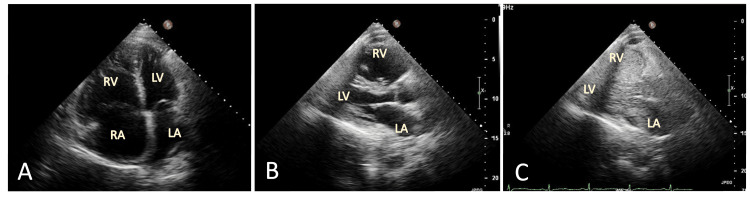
Initial TTE evaluation (A) Apical 4-chamber view. (B) Parasternal long axis view. (C) Demonstrating markedly enlarged right heart structures with marked right-to-left shunting of agitated saline. TTE: transthoracic echocardiogram; RV: right ventricle; RA: right atrium; LV: left ventricle; LA: left atrium.
